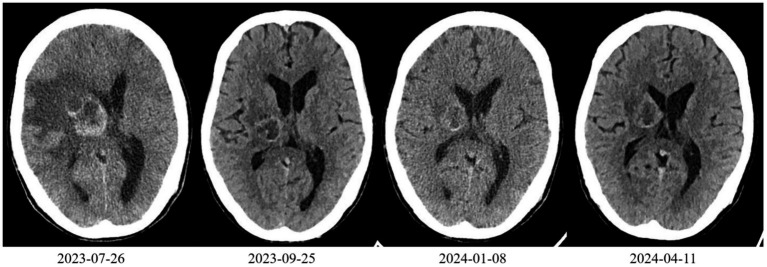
Cranial CT scan of the patient.
